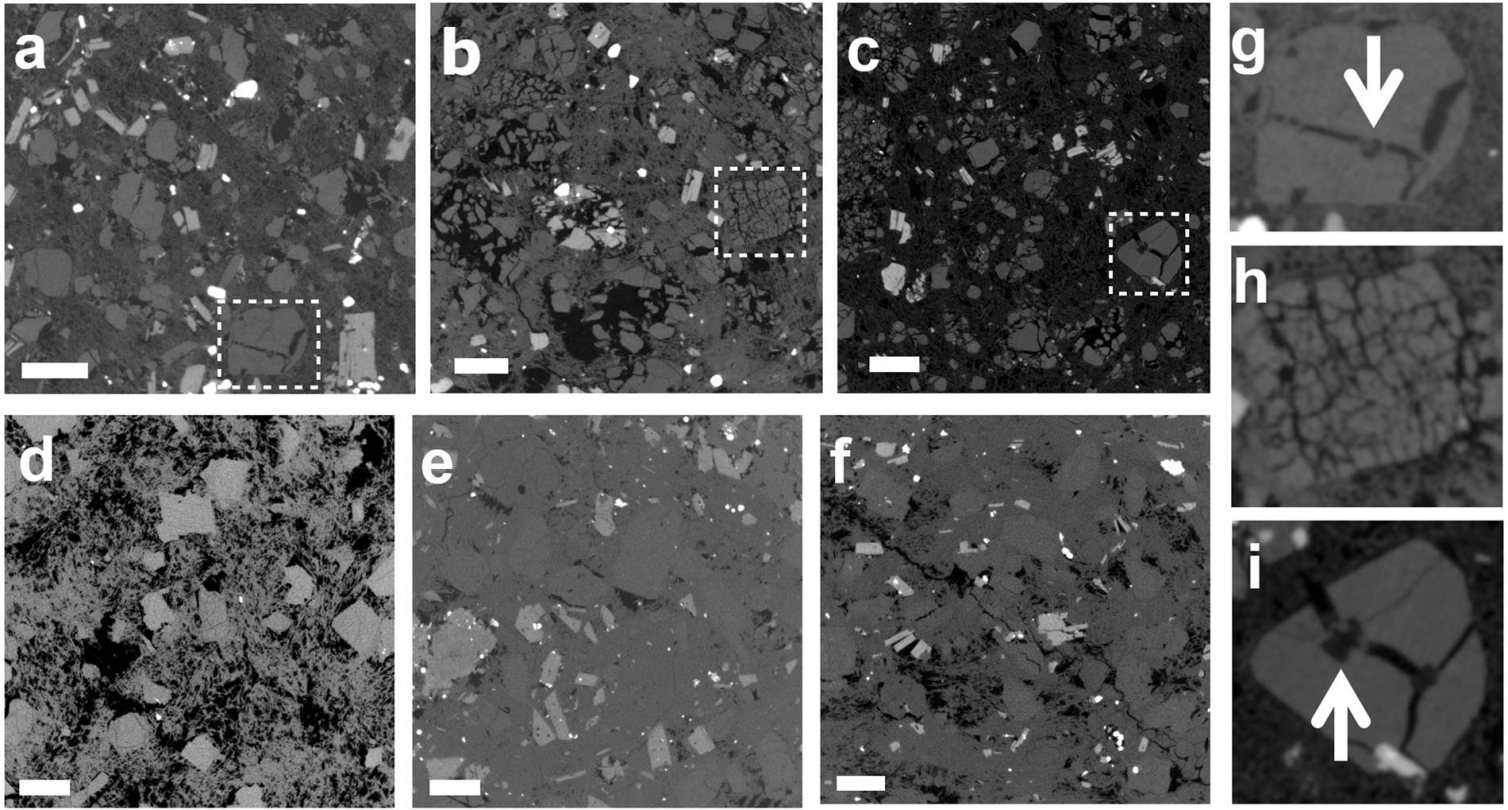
Typical CT images of pumices (a, 07BOL23PD; b, 07BOL011; c, 83019) and lavas (d, 07CB012A; e, 09005CT; f, 09011CT). The images of (g–i) show broken phenocrysts, which correspond to the areas enclosed by white dotted lines in (a–c), respectively. The black portions represent pores/vesicles. The grey portion corresponds to felsic minerals and groundmass. The light grey and white particles are mafic minerals. Foamed melt inclusions are found along fractures inside the phenocrysts in (g,i) (white arrows). (h) Represents an example of phenocryst fragmentation without foamed melt inclusion. The white bars represent 1 mm scale.
